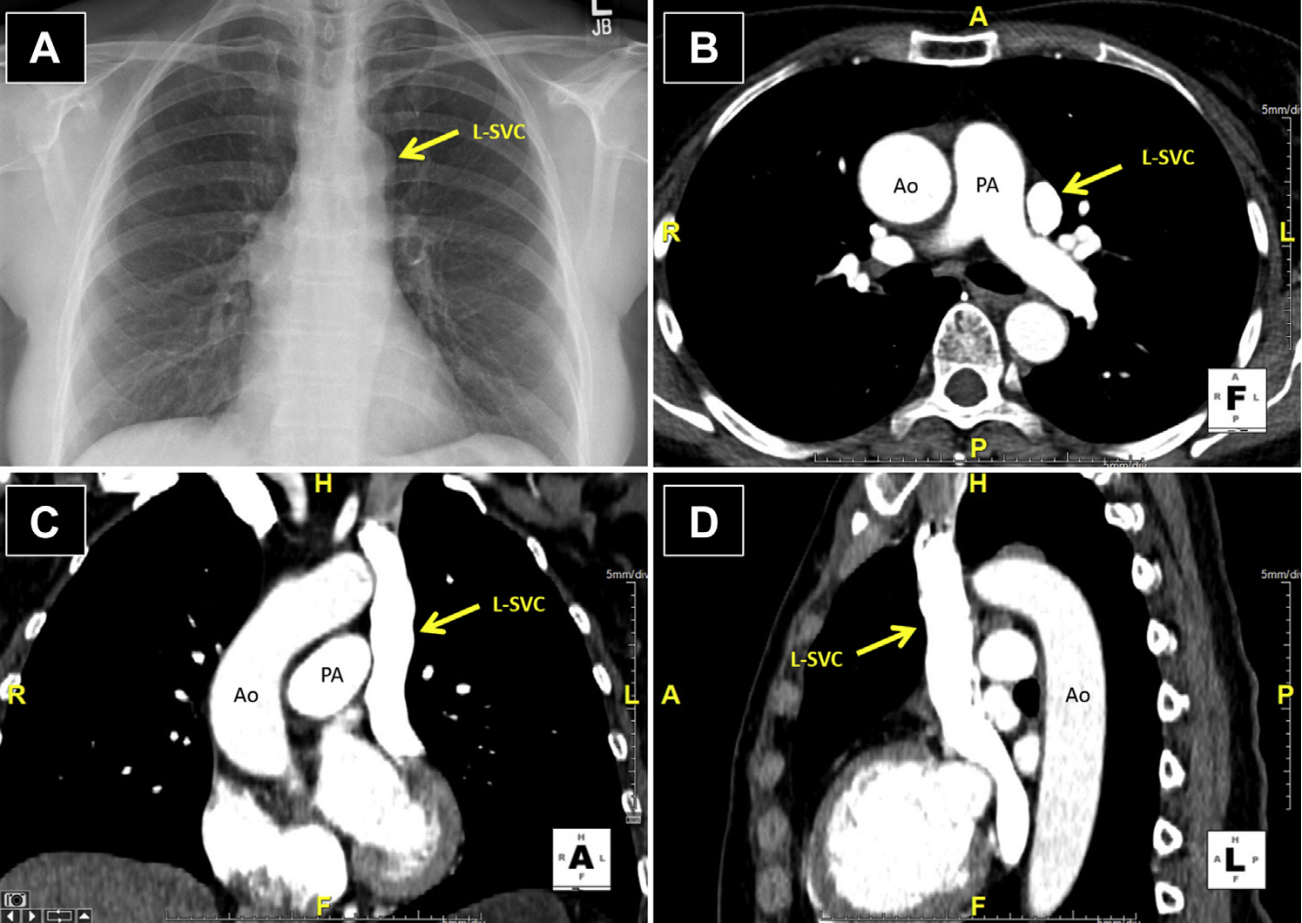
Case 1: persistent L-SVC. Chest x-ray with anteroposterior projection and CCT with axial, coronal, and sagittal views. A widened mediastinum on chest x-ray (A) was initially concerning for aortic dissection (*arrow*), but subsequent CCT imaging in this patient identified it as a persistent L-SVC (B-D). *Ao*, Aorta; *PA*, pulmonary artery.
